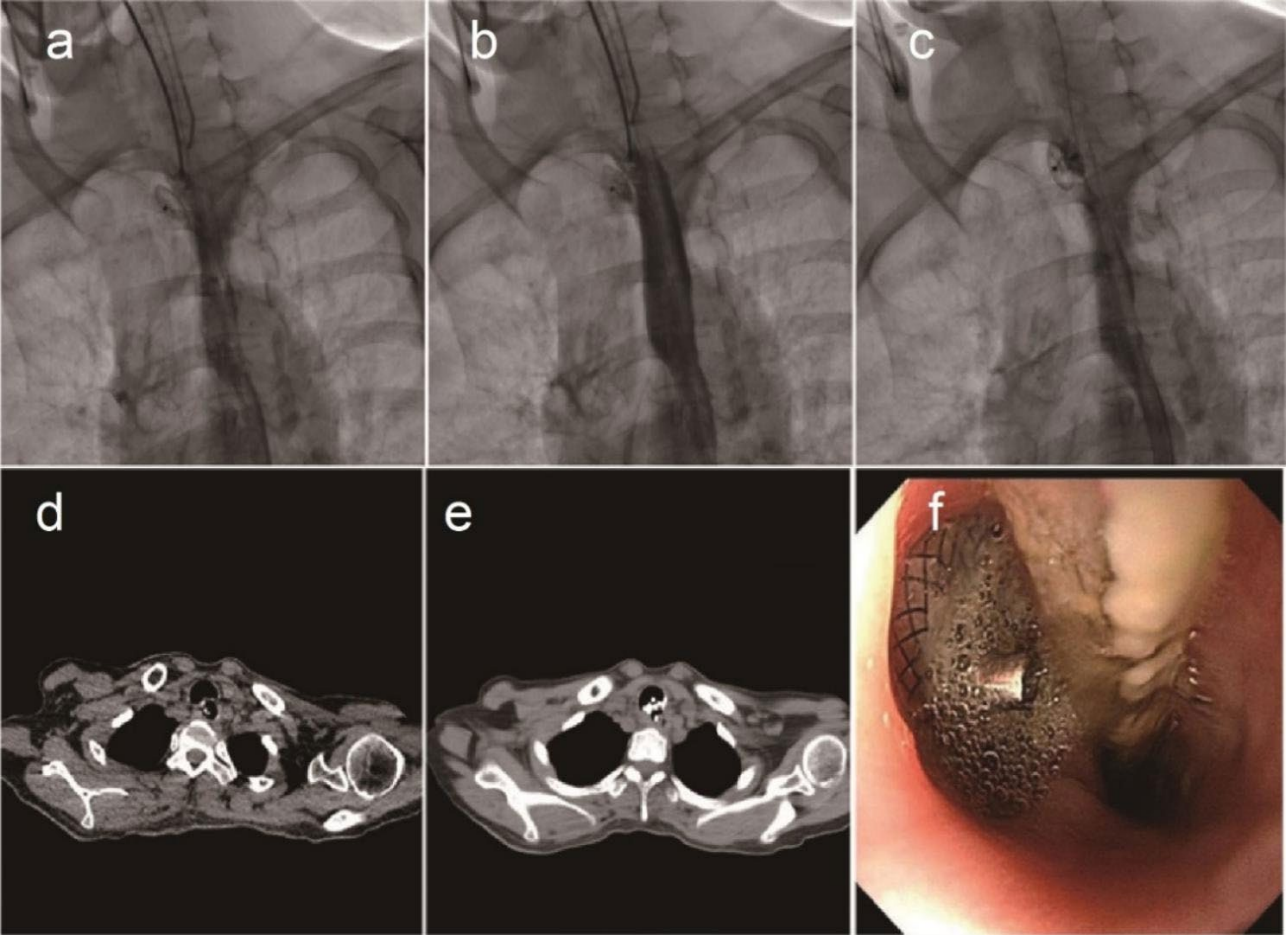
ASD placement to treat an ETF that failed to be cured by oesophageal stenting. The distal disk was released into the trachea (a). The proximal disk was released into the oesophagus. The oesophagogram via a catheter demonstrates the successful occlusion of the ETF (b). The ASD was detached and left in place to occlude the ETF (c). Representative CT image showing the fistula before (d) and after (e) ASD placement. Representative oesophagogastroscopy image of AD
